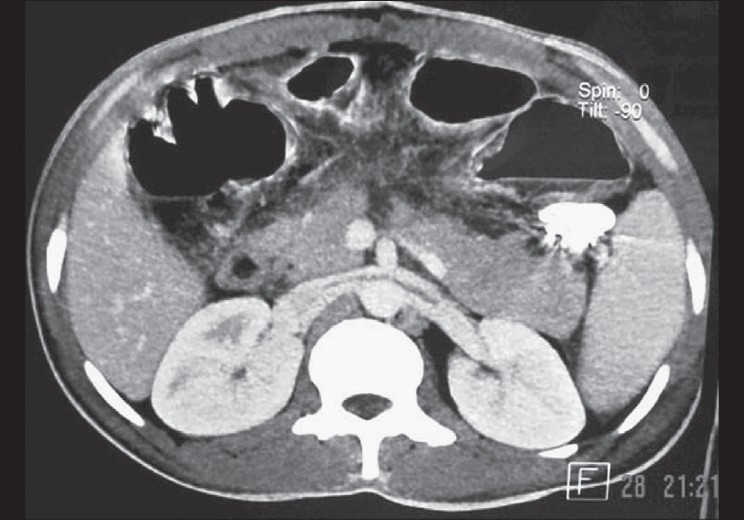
Axial CT image shows complete fracture through the neck of the pancreas, minimal peripancreatic fluid and soft tissue stranding in the peripancreatic fat
